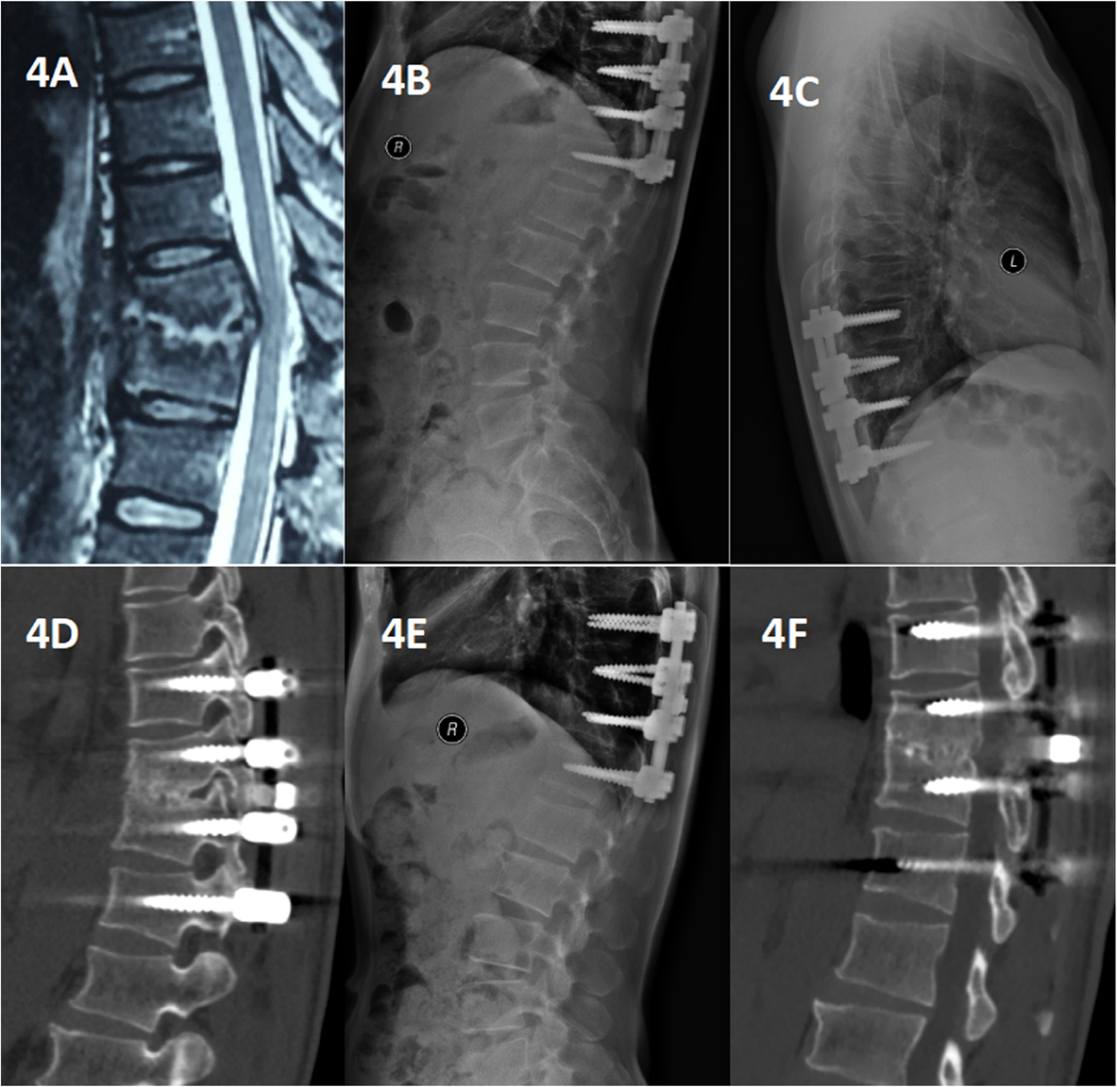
A 40-year-old man with thoracic spinal tuberculosis (T10-11) underwent posterior debridement and decompression combined with internal fixation. (a) Pretreatment MRI showing destruction of the T10-11 vertebrae and concomitant compression of the spinal cord. (b,c,d) Immediate postoperative and 6-month radiographs and 6-month CT demonstrating posterior debridement, bone graft and internal fixation. (e, f) At the 22-month follow-up, plain X-ray and CT showed maintenance of the correction and solid fusion
